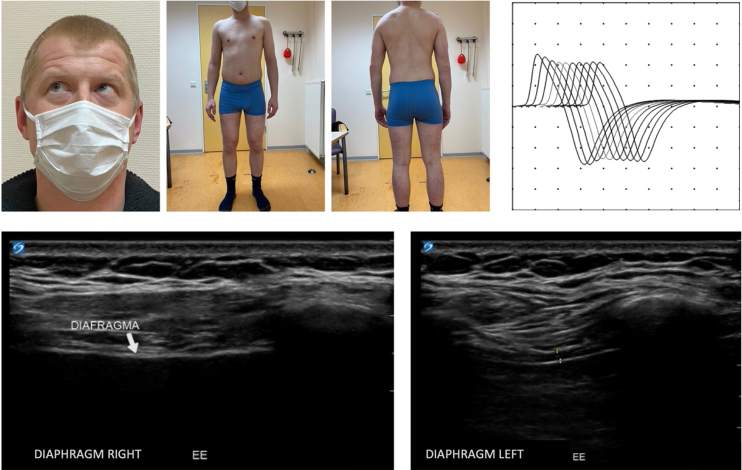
Top left: Clinical photographs, taken at the age of 32, showing a mild ophthalmoplegia of the right eye with upward-outward gaze and a lack of muscular atrophy. Top right: EMG with RNS showing decrement of CMAP amplitude of the trapezius muscle. Bottom: Ultrasound of right and left side of the diaphragm. The right side of the diaphragm shows severe muscle atrophy and no thickening during inspiration. The left side shows no abnormalities. The thickness at end-expiration is 1.7 mm and thickening ratio during inspiration is 3.1.
